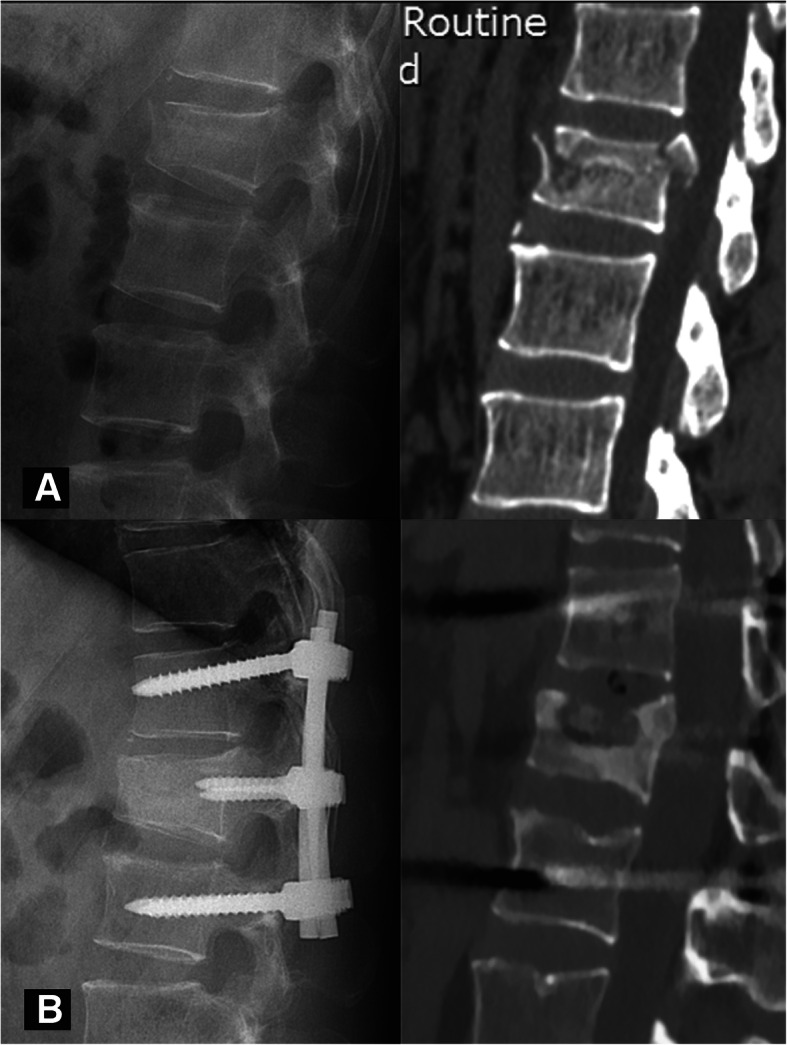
A 52-year-old male had a burst fracture at L1, with an ASIA grade for neurological function of E on admission. a Preoperative X-ray and CT images show an A3 burst fracture. b Postoperative X-ray and CT images at 18 months show a large oval cavity in the superoanterior part of the vertebral body that was larger than 1/3 of the vertebral body volume, which was classified as HPC type III
